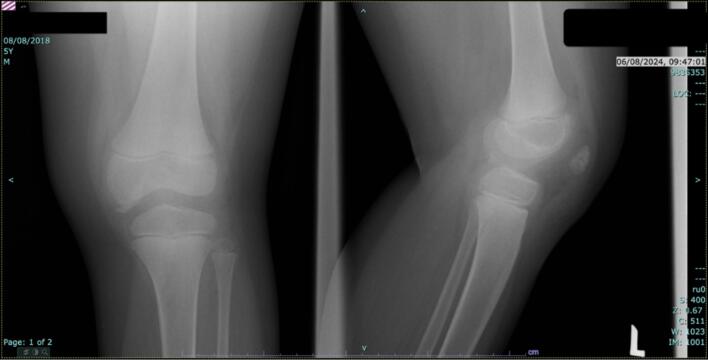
One-year postoperative plain radiographs of the left knee (AP and lateral views).
